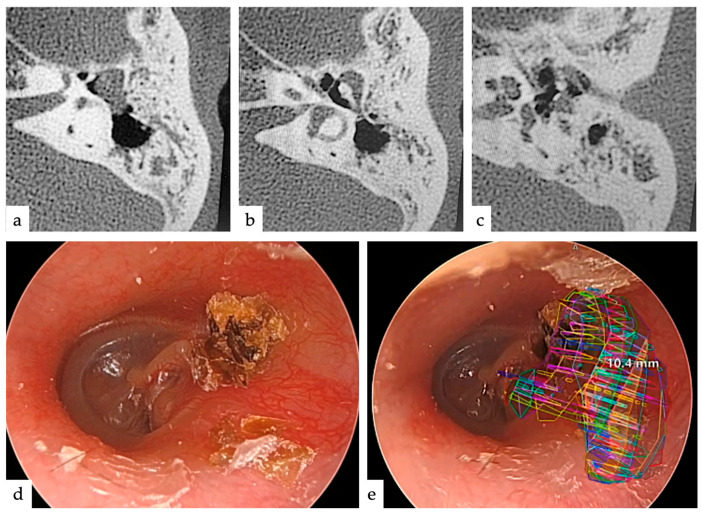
Imaging findings and AR display in Case 2. The AR display shows that the area of EAC drilling to be performed is large, whereas the area of progress is not very large. (a) Axial CT slice of the temporal bone showing a slight extension into the antrum. (b) Axial CT slice of the temporal bone showing extension into the attic. (c) Axial CT slice of the temporal bone showing extension into the tympanic cavity. The lesion extended close to the stapes; however, the superstructure did not exhibit any erosive changes. (d) Typical endoscopic findings of cholesteatoma with debris in the pars flaccida. (e) Endoscopic image with AR display overlaid on image (d). Extensive EAC drilling was required to remove the lesions. AR: augmented reality; EAC: external auditory canal; CT: computed tomography.
